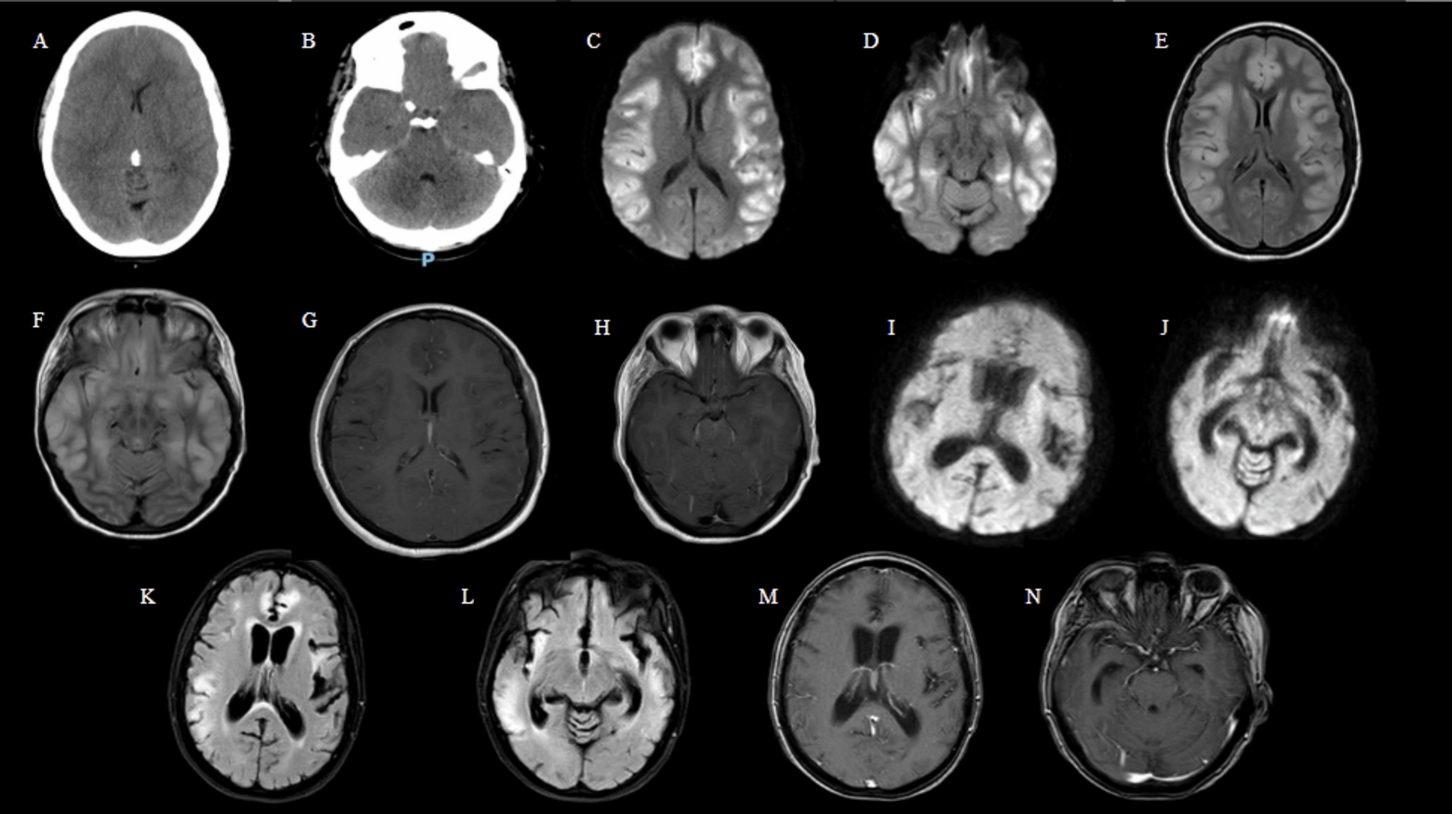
Neuroimaging findings of Case 1. (A, B) CT head scan obtained on hospital day 1, showing global cerebral edema and brainstem compression at the midbrain level; (C-H) MRI brain scan obtained on hospital day 12: (C, D) diffusion-weighted imaging (DWI) showing extensive hyperintensities in the cerebral cortex; (E, F) T2 Fluid-Attenuated Inversion Recovery (T2-FLAIR) sequence showing hyperintensity changes in the corresponding areas of DWI abnormalities; and (G, H) post-contrast axial imaging showing leptomeningeal enhancement consistent with encephalitis. (I-N) Follow-up MRI brain scan obtained on day 75 after admission: (I, J) DWI showing improvement in cortical hyperintensity changes; (K, L) T2-FLAIR sequences of corresponding sections demonstrating predominant limbic region hyperintensities with concurrent brain atrophy and ex vacuo dilatation of the lateral and third ventricles; and (M, N) post-contrast axial imaging showing improving sequelae of limbic encephalitis.
